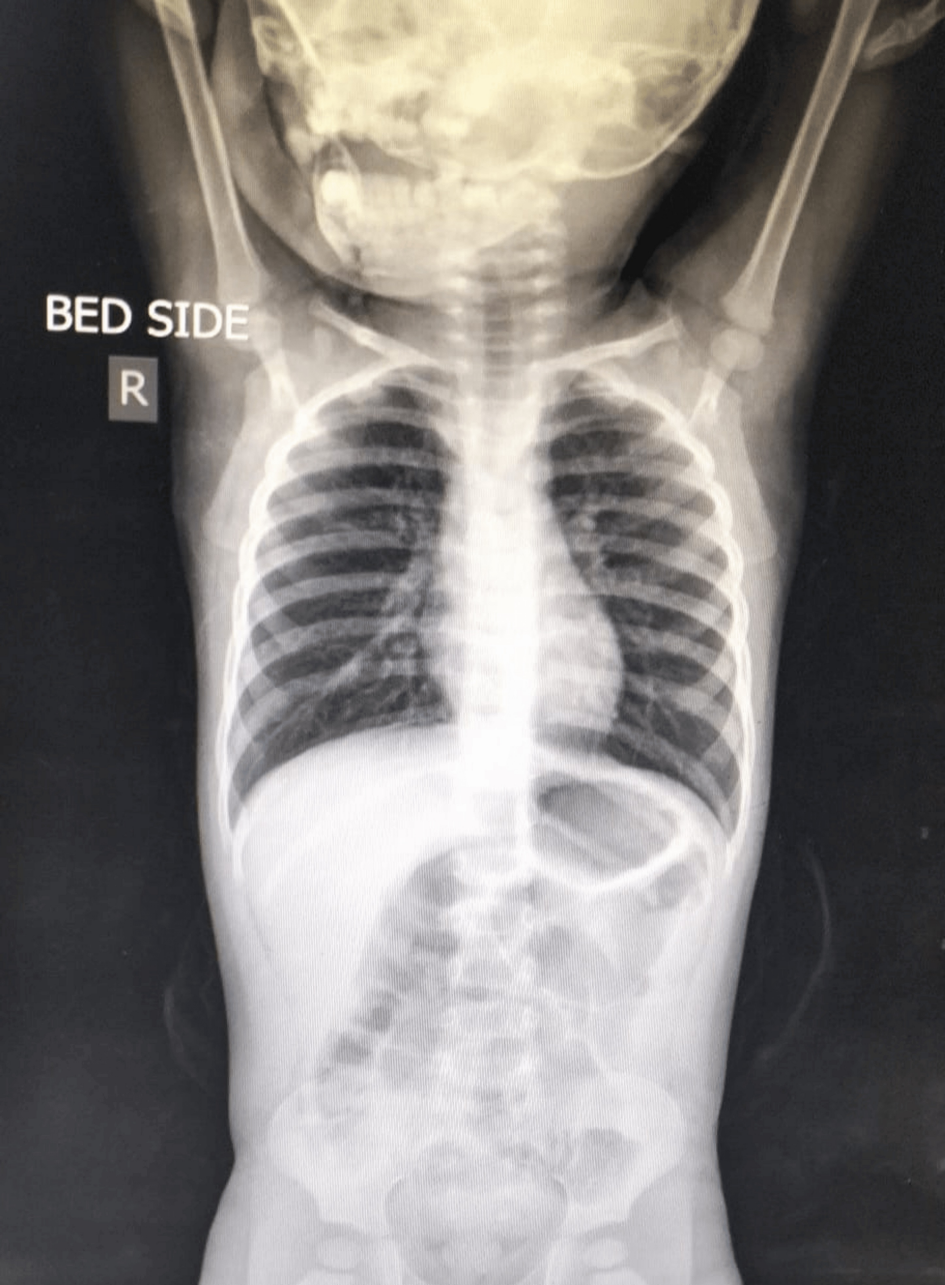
Chest X-ray showing bilateral hyperinflated lung fields
